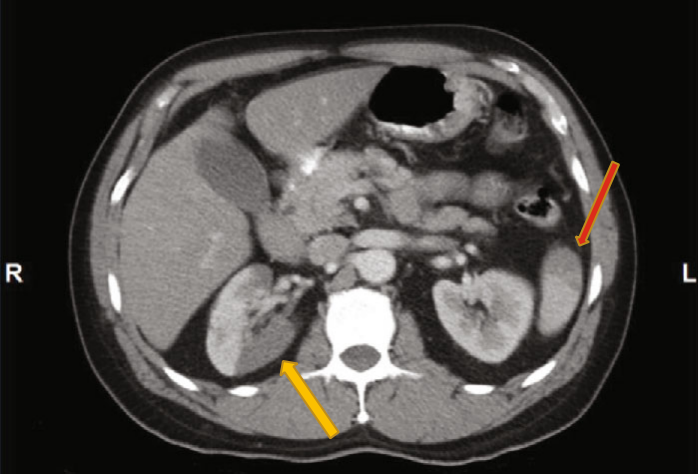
A CT scan of the abdomen: yellow arrow - right renal infarction; red arrow - splenic infarction.
